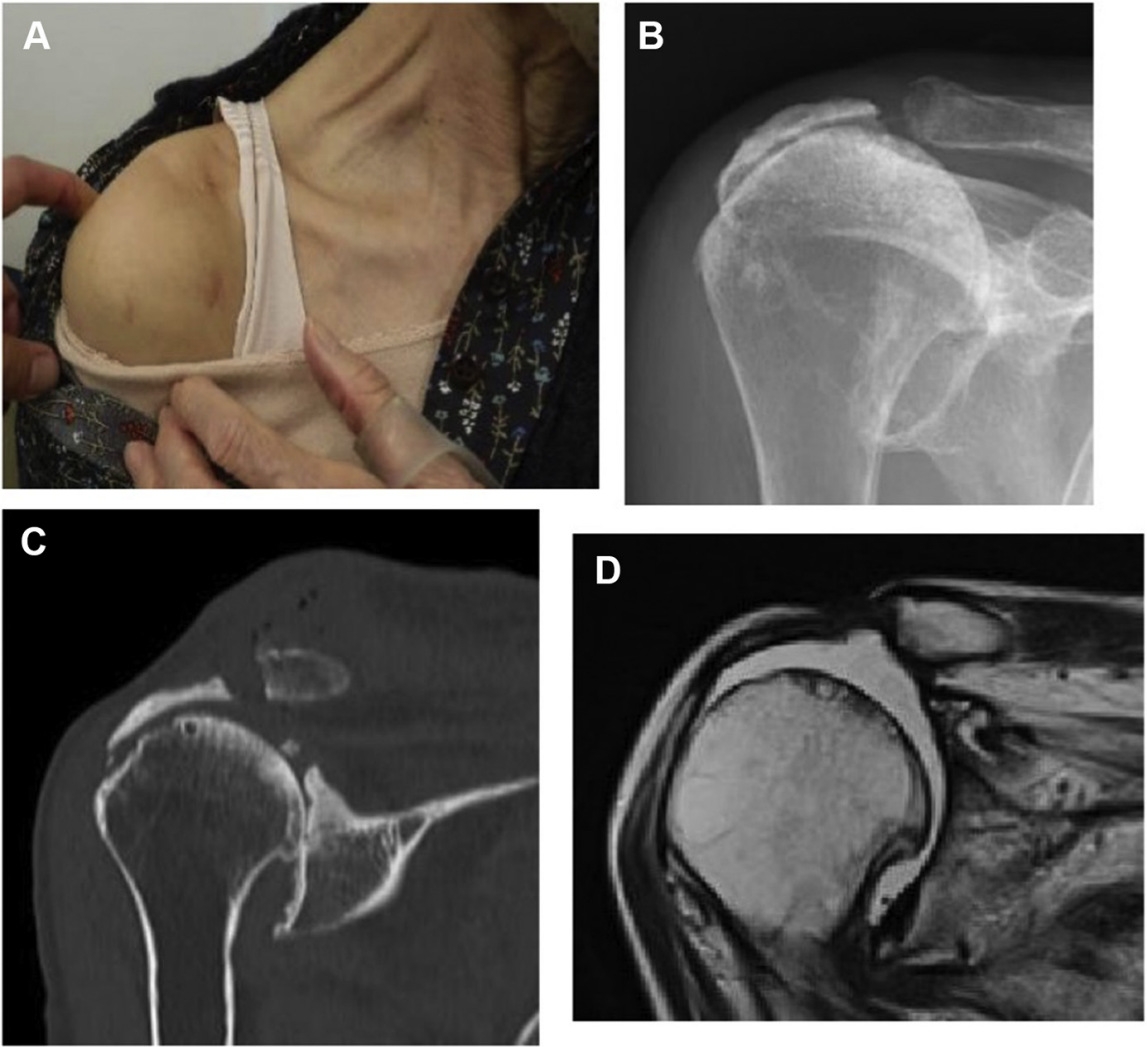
Postoperative swelling of the acromioclavicular joint and postoperative images of Case 2. (A) The swelling of the acromioclavicular joint has disappeared. (B, C) Approximately 3 mm is resected from the distal end of the clavicle. (D) MRI scan shows that the cyst in the superior section of the acromioclavicular joint has disappeared. *MRI*, magnetic resonance imaging.
